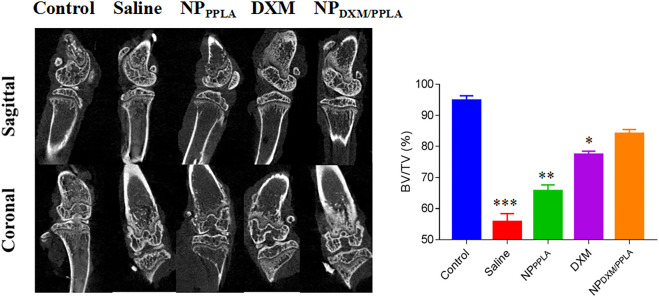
The efficacies of NS, NP_PPLA_, DXM and NP_DXM/PPLA_ on OA were observed by Micro CT and analyzed the bone volume fraction (BV/TV) in subchondral region.
